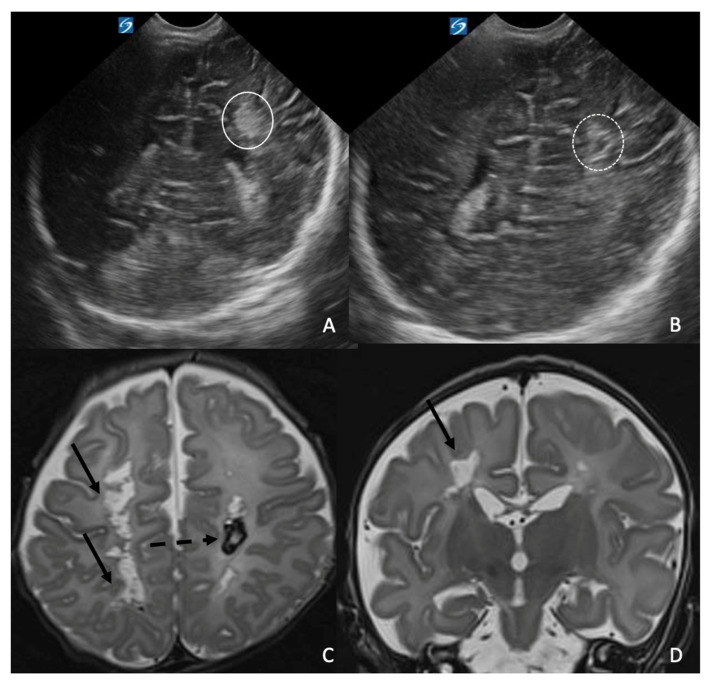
Brain USs performed at 10 and 20 days of life and the brain MRI performed at 1 month of age in a girl diagnosed with *Listeria monocytogenes* infection. The coronal US Images show the presence of a hyperechoic hemorrhagic focus (white circle in (A)), which showed a subsequent hypoechoic core, consistent with progressive resorption (dotted white circle in (B)). The axial (C) and coronal (D) T2WI sequences show multiple bilateral periventricular and centrum semiovale cystic lesions (black arrows) distributed along the course of the deep medullary veins, some of which demonstrate the presence of blood products (dotted black arrow in (C)).
